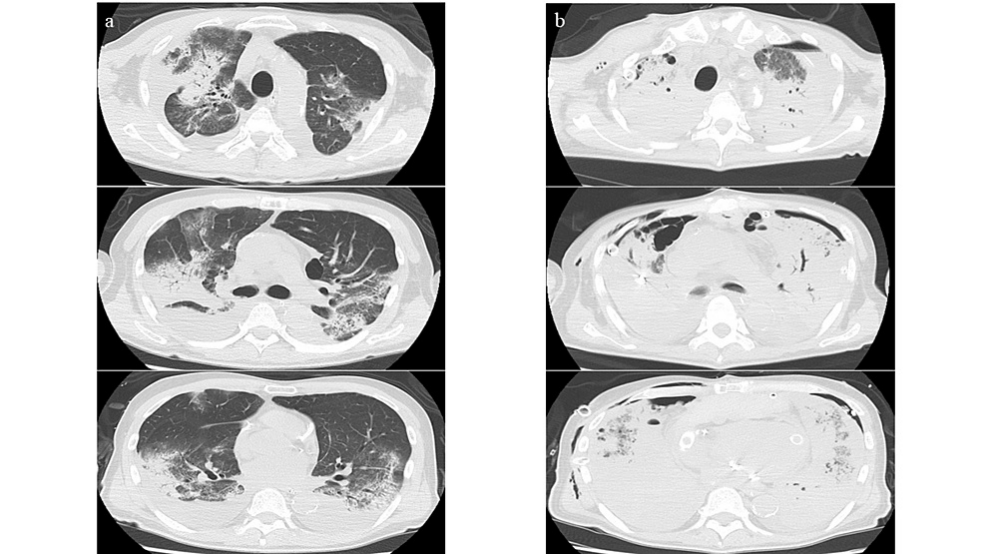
Changes in chest CT findings a; before Impella 5.0, b; after extubation on hybrid VA-ECMO CT; computed tomography, VA-ECMO; veno-arterial extracorporeal membrane oxygenation
